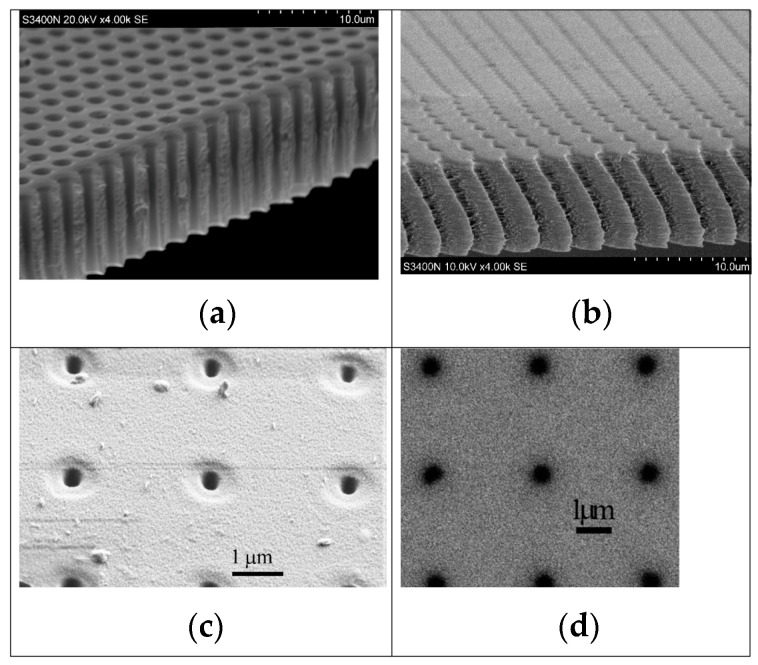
(a–c) SEM images of channels fabricated in PET film using X-ray lithography and (d) X-ray mask. Copyright 2021 IOP Publishing. Reproduced with permission from [96]. All rights reserved.
